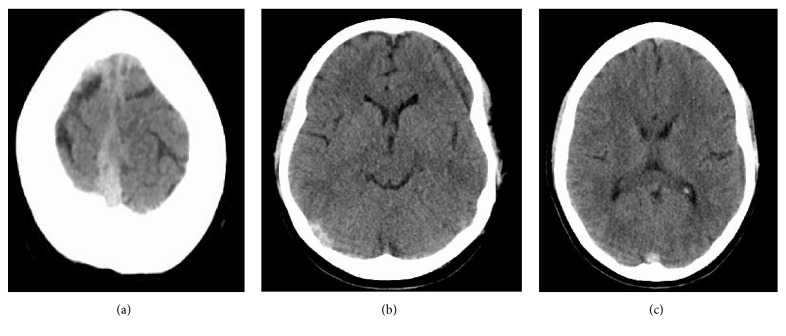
Initial head CT without contrast in our patient described above noted a falcine with bifrontal subdural hematoma at the vertex (a). Note the hyperdense right transverse sinus (b) and “filled delta sign” (c).
